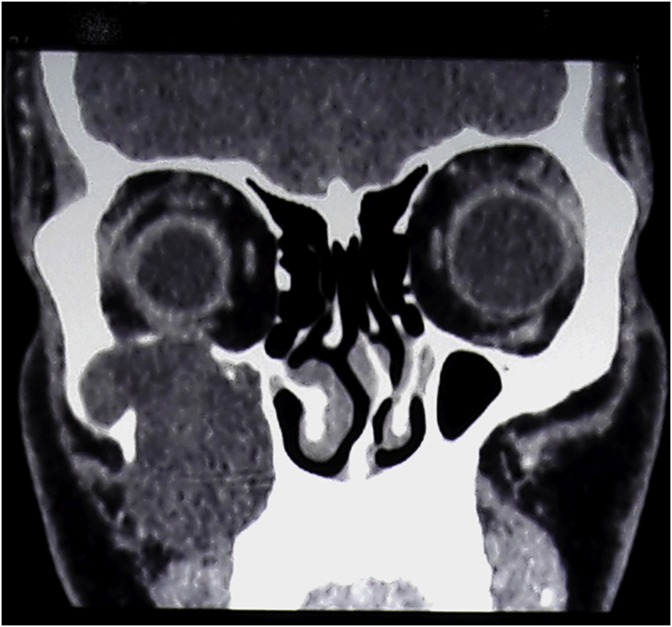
CT showing hypodense lesion in right maxillary sinus.
